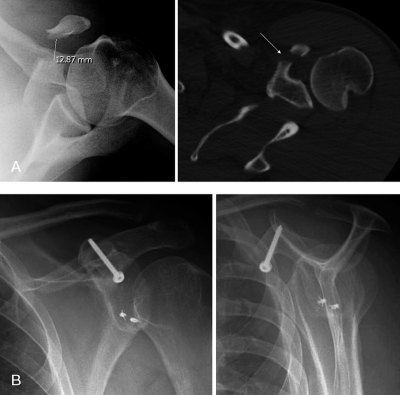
A. AP radiograph (left) and CT scan of the scapula (right) showing fracture of the middle coracoid with > 1 cm of displacement. B. AP (left) and lateral (right) radiographs after open reduction and internal fixation using a cortical lag screw.
